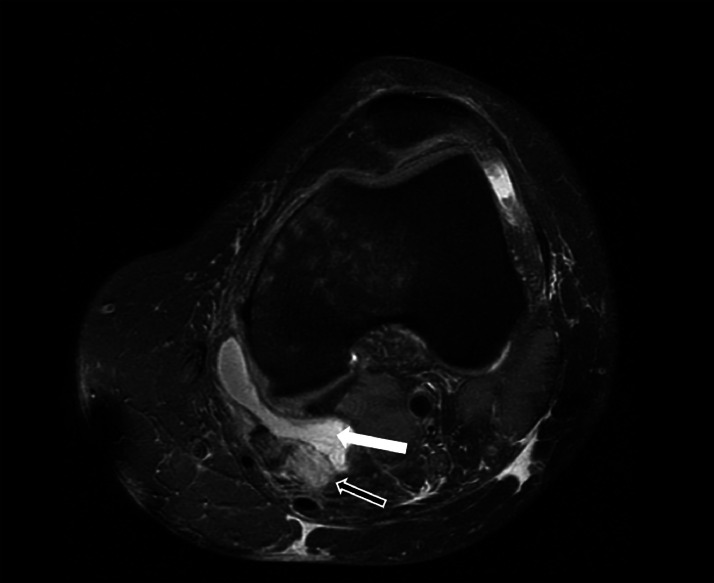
T2-weighted axial image at presentation. The black arrow demonstrates the retracted distal end of the semimembranosus tendon. The white arrow demonstrates the fluid filled space secondary to the rupture.
